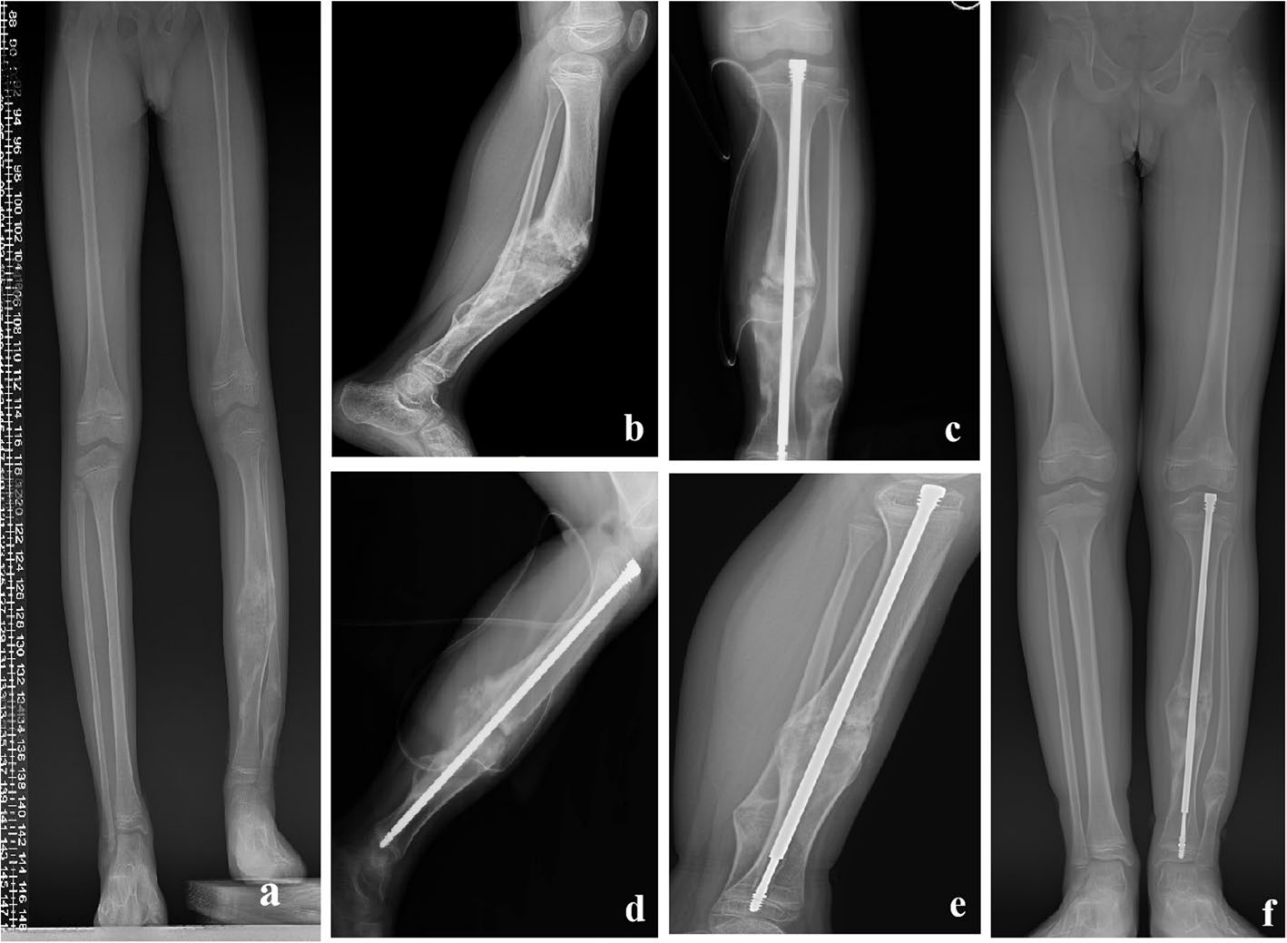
Outcomes for an 11-Year-Old Boy with OFD of the Left Tibia. a, b Anteroposterior (a) and lateral (b) radiographs obtained preoperatively, indicating a 48-degree anterior bowing deformity in the lateral view. c, d Intraoperative anteroposterior (c) and lateral (d) radiographs, displaying the wo tibial osteotomies performed. e,f, g Radiographs taken two years postoperatively, revealing sound bone healing, absence of deformity, and no implant failures. c, d, e, f the specific details of the distal tip of the nail in the postoperative and 2-year follow-up images,4-mm prolongation was present
